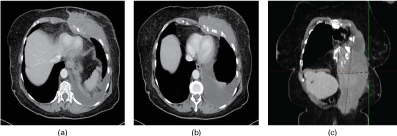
CT axial (a,b) and coronal (c) views demonstrating large soft-tissue mass extending into rectus abominus, with associated rib destruction, pleural effusions and restriction of the left hemithorax.
